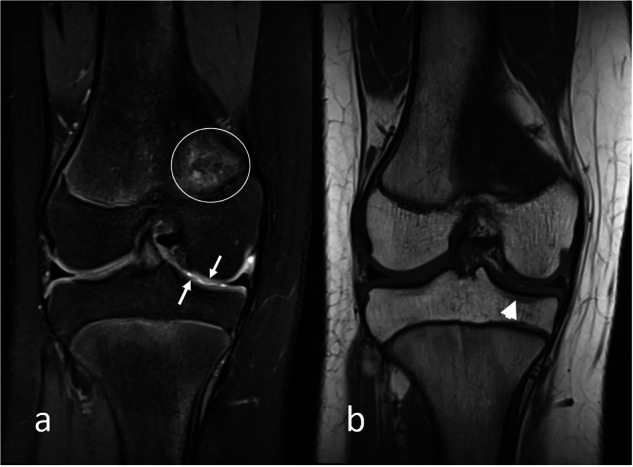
Knee MRI in an 11-year-old girl with JIA, (a) coronal PD-weighted fat-saturated image showing irregular cartilage in the femorotibial medial compartment (arrow) and (b) subtle subchondral sclerosis on a coronal T1-weighted image (arrowhead). Note the oedema in the distal medial femoral metaphysis, representing a cortical desmoid (circle)
